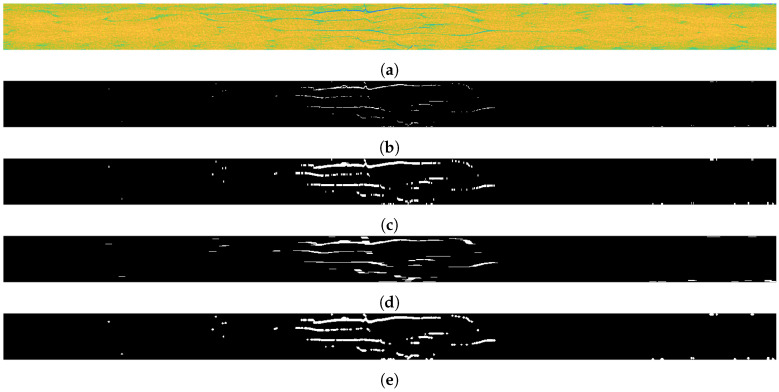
Processing of the results: (a) original CT slice; (b) recognized cracks; and directional expansion in the direction: (c) vertical; (d) horizontal; (e) diagonal.
